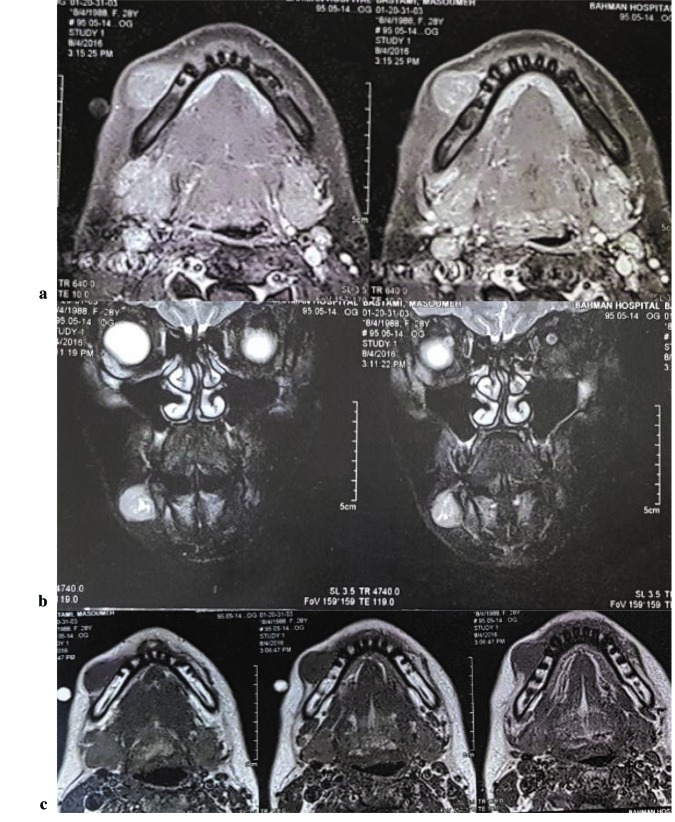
MRI scans show a well-defined soft tissue mass in the right side of mandible. a: T1 without Gadolinium, b: T1 with Gadolinium, c: T2 with Gadolinium
